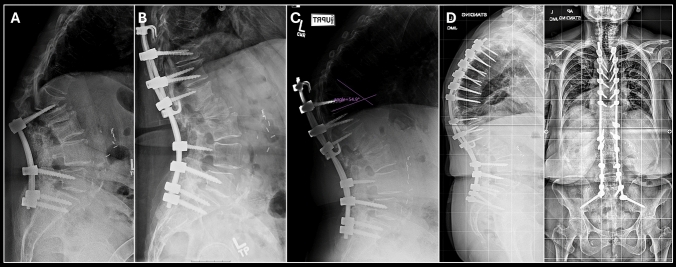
A 74-year-old female with a history of L2–S1 posterior lumbar fusion presented with acute low back pain, and inability to walk. A Preoperative lateral X-ray demonstrated L2 pedicle screw pullout and kyphotic deformity above the fusion construct due to a fracture dislocation at L1/2. B Patient underwent revision T12–L3 laminectomies and L2 transpedicular decompression with open reduction of kyphotic deformity and T10–S1 posterolateral lumbar fusion with postoperative lateral X-ray shown. C Three months postoperatively, the patient presented with worsening pain, kyphosis and a power of 3/5 with lateral X-ray showing new acute thoracic kyphosis. D Patient underwent revision T4–ilium posterolateral arthrodesis and instrumentation. Postoperative lateral and posterior–anterior X-rays are shown. Postoperatively, the power was 1/5 and improved to 4/5 at the last clinical follow-up
